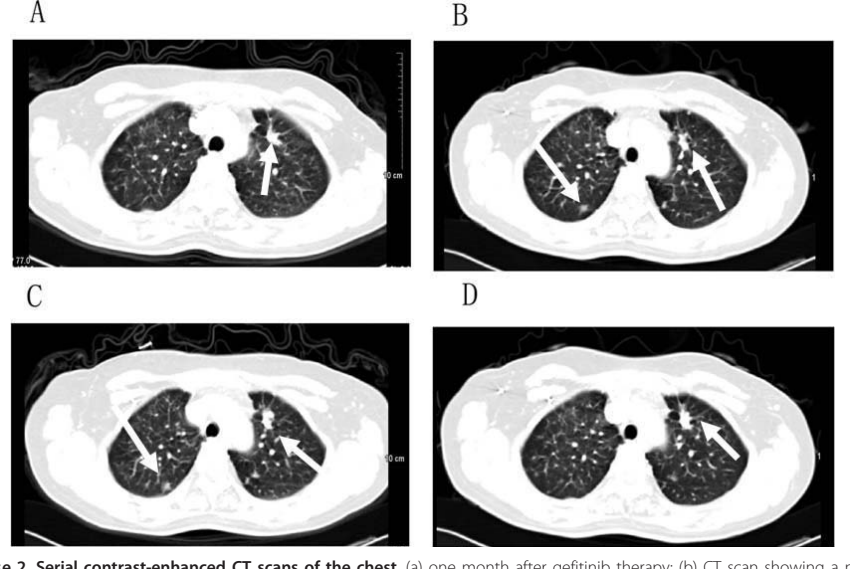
Case 2. Serial contrast-enhanced CT scans of the chest. (a) one month after gefitinib therapy; (b) CT scan showing a new lesion at the right lund after 29 months of gefitinib therapy; (c) CT showing a stable disease of the lung nodule after two cycles of chemotherapy; (d) CT scan showing a marked regression of the tumor in the right lung, and stable disease of the tumor in the left lung after one month of gefitinib re-challenge.
